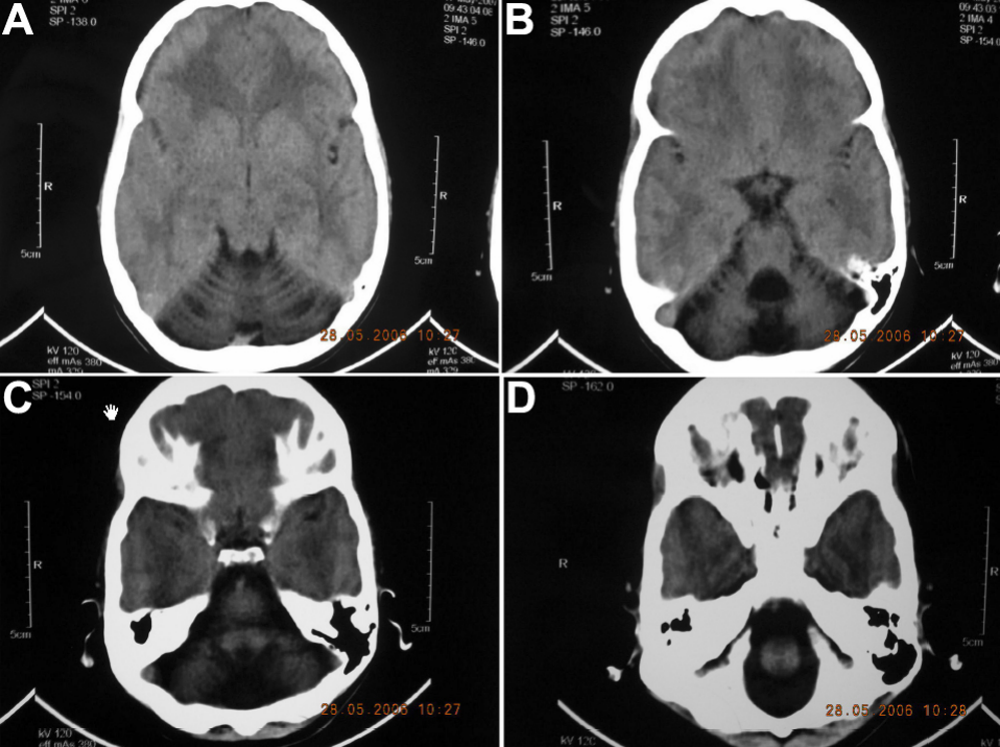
Computed tomography (CT) scan results of individual 09, family 60078. A depicts cerebellar dysgenesis/atrophy, reduced density of cerebellar cortex and atrophy of superior vermis, while B shows dilation of the 4th ventricle, lack of communication between 4th ventricle and Cisterna Magna and absence of Posterior inferior part of vermis. C shows the absence of inferior limit of vermis and cerebellar cortical atrophy and D demonstrates reduced density of the cerebellar cortex.
